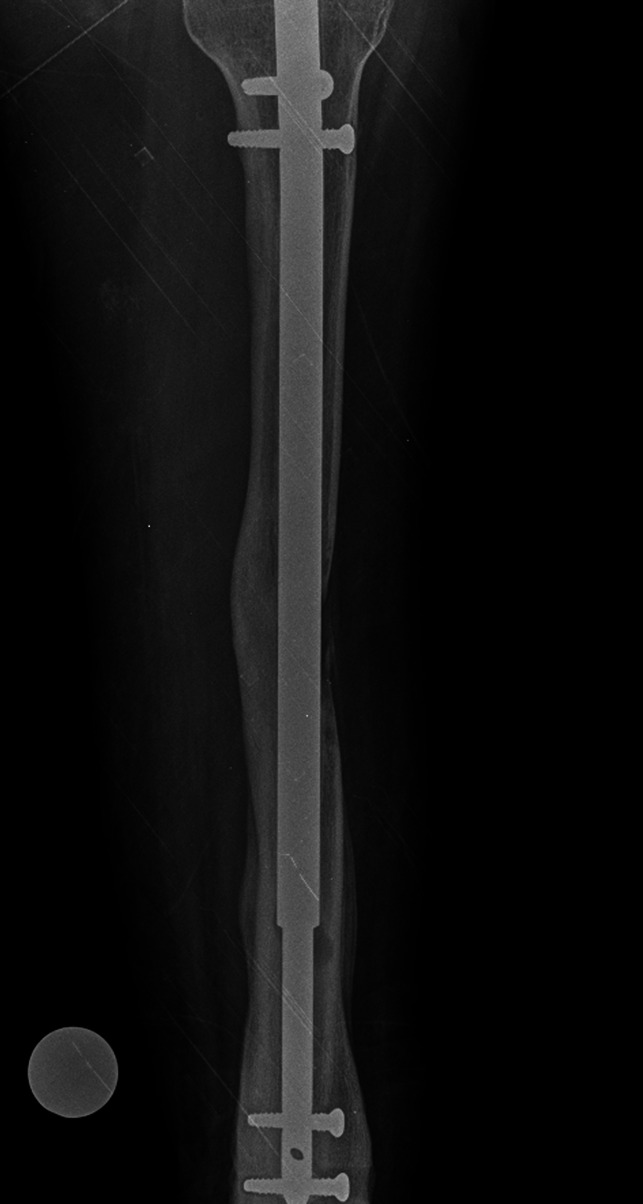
AP radiograph of a right tibia demonstrating a punched-out appearance distal and medial to the modular junction resembling osteolysis.
